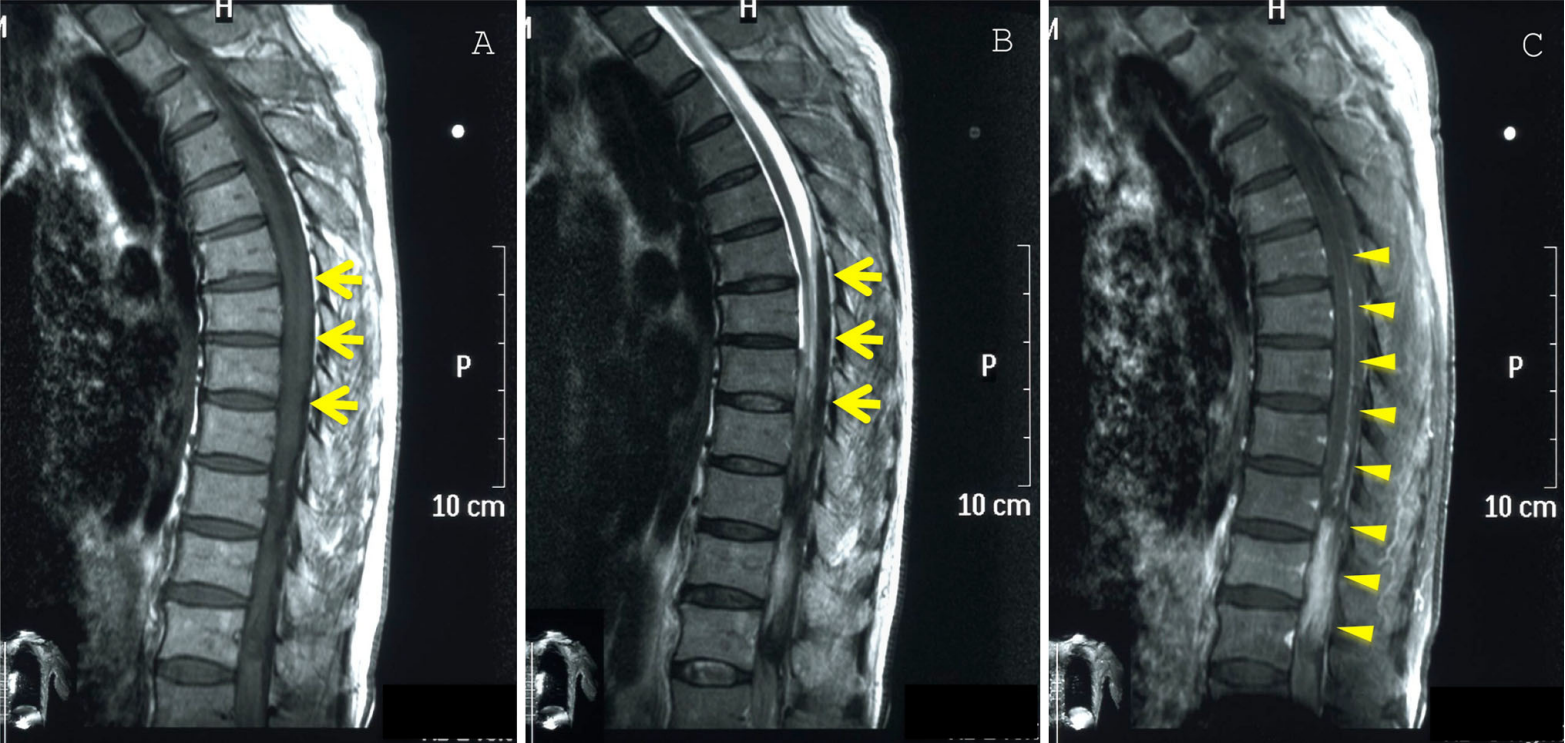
MRI of the thoracic spine. Sagittal T1-weighted MR image (a), T2-weighted MR image (b), and T1-weighted MR image with contrast enhancement (c). a Fluid–fluid level was seen in the dorsal side of the spinal cord as a slightly high intensity signal (*arrows*). b Fluid–fluid level was seen in the dorsal side of the spinal cord as a low-intensity signal (*arrows*). c An intradural extramedullary enhancing mass was seen after intravenous administration of gadolinium diethylenetriaminepentaacetic acid (*arrow heads*). This indicated drop metastases (*arrow heads*) with hemorrhage (*arrows*)
